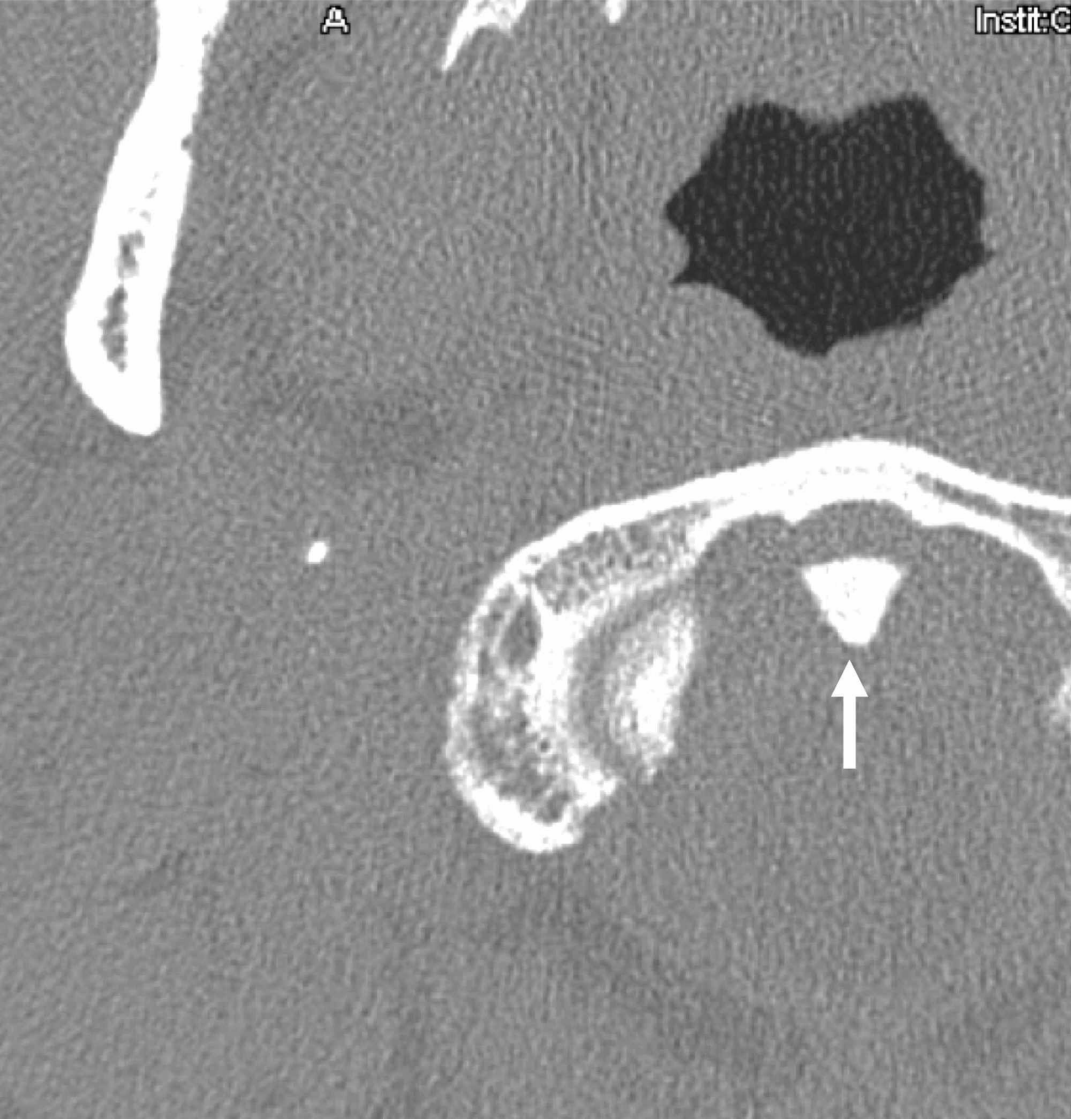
Axial CT of the skull in the case Note the triangular-shaped odontoid process (arrow). CT: computed tomography
